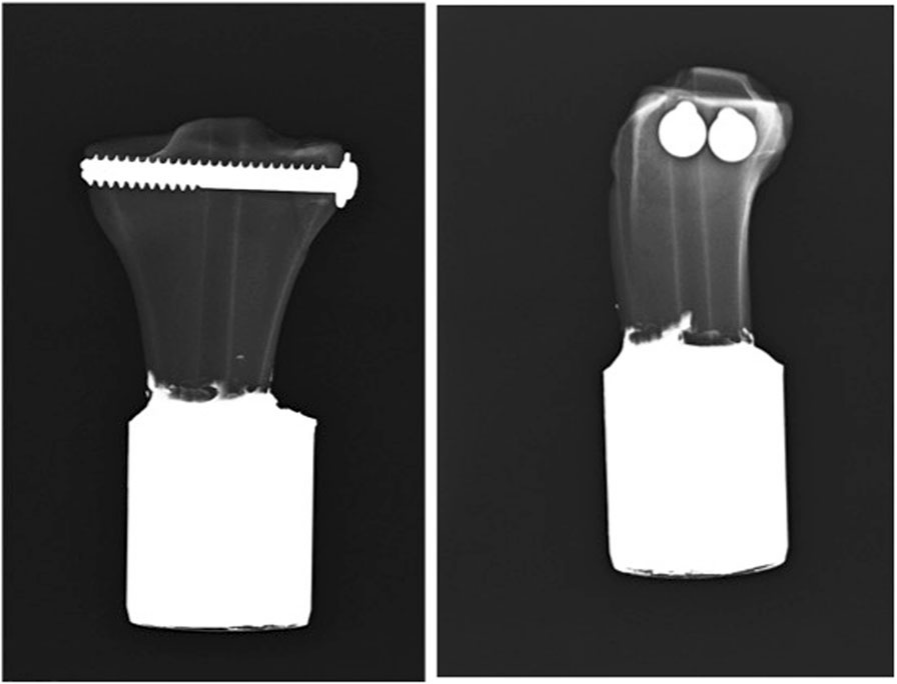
AP and lateral X-rays of the bone model after screw fixation
